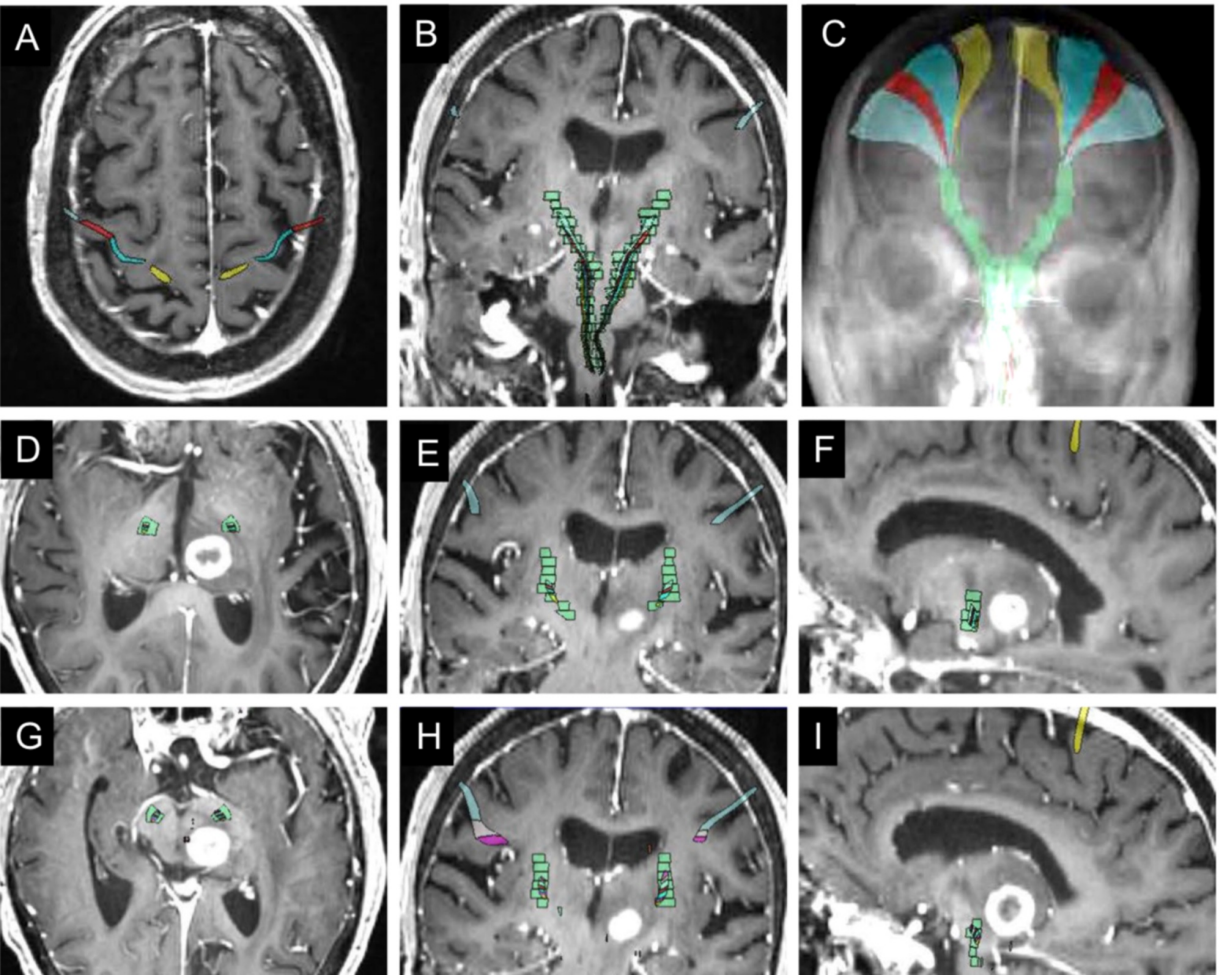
Proximity of target metastasis to corticospinal tracts (CST) using co-registration of diagnostic MRI and Anatom-e atlas (A-C) Patient diagnostic MRI merged to Anatom-e defined motor cortex (A) and CST (B) with 3D somatotopic rendering of motor fibers coalescing into the CST (green) (C). (D-I) Co-localization of target lesion with CST (green) in axial (D, G), coronal (E,F) and sagittal (F,I) planes.
